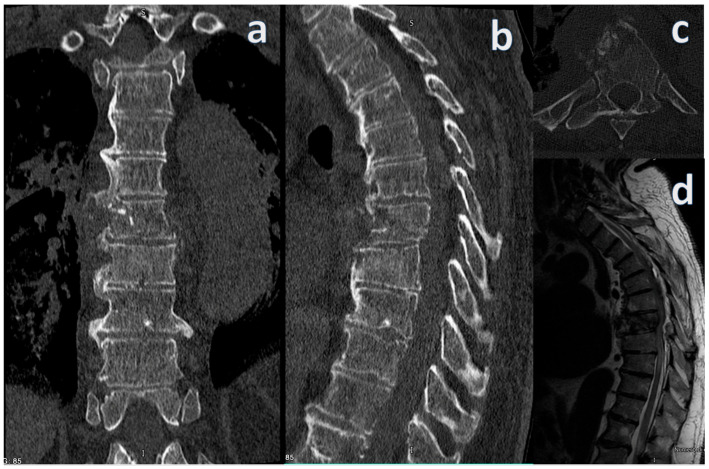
70-year-old male patient affected by D7 fracture (AO type B) and spinal cord injury ASD. (a) CT scan coronal view (b) CT scan lateral view (c) CT scan axial view (d) MRI lateral view.
